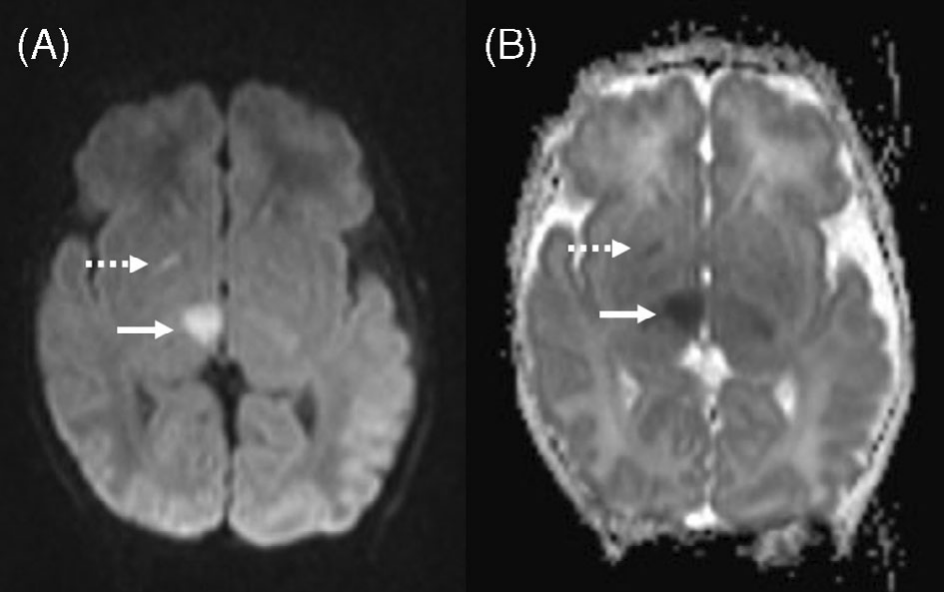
MRI of patient 1 at the age of 3 days: (A) diffusion weighted image and (B) ADC map show focal diffusion restriction in right basal ganglia (dashed arrow) and right thalamus (solid arrow).
